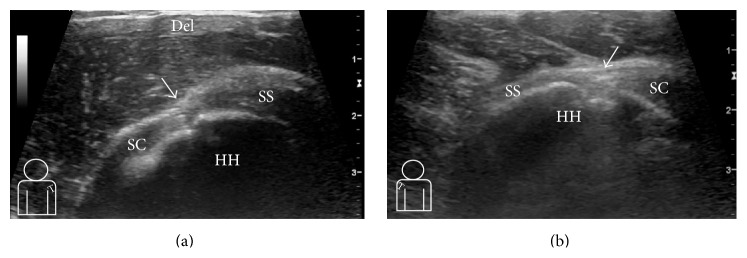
Ultrasound (US) images of both shoulders. (a) US parasagittal image of the left shoulder rotator interval. The long head of the biceps tendon is not identifiable in its normal position (white arrow). (b) US parasagittal image of the right shoulder rotator interval. The long head of the biceps tendon is not discernible in its normal position (white arrow). HH = humeral head; SS = supraspinatus tendon; SC = subscapularis tendon; Del = deltoid muscle.
